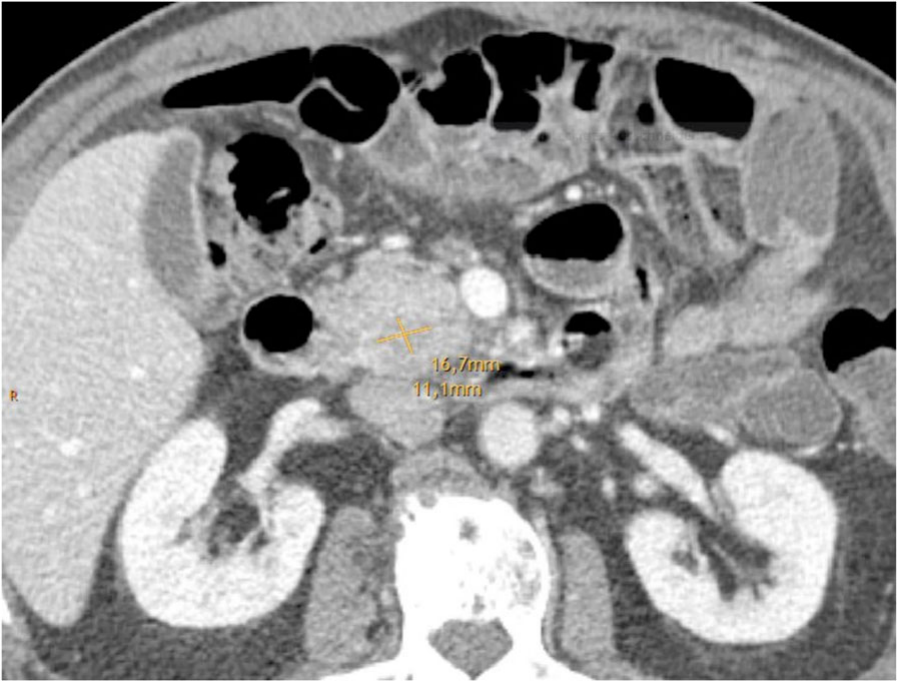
Contrast-enhanced CT in portal venous phase shows the hypodense lesion in the pancreatic head
